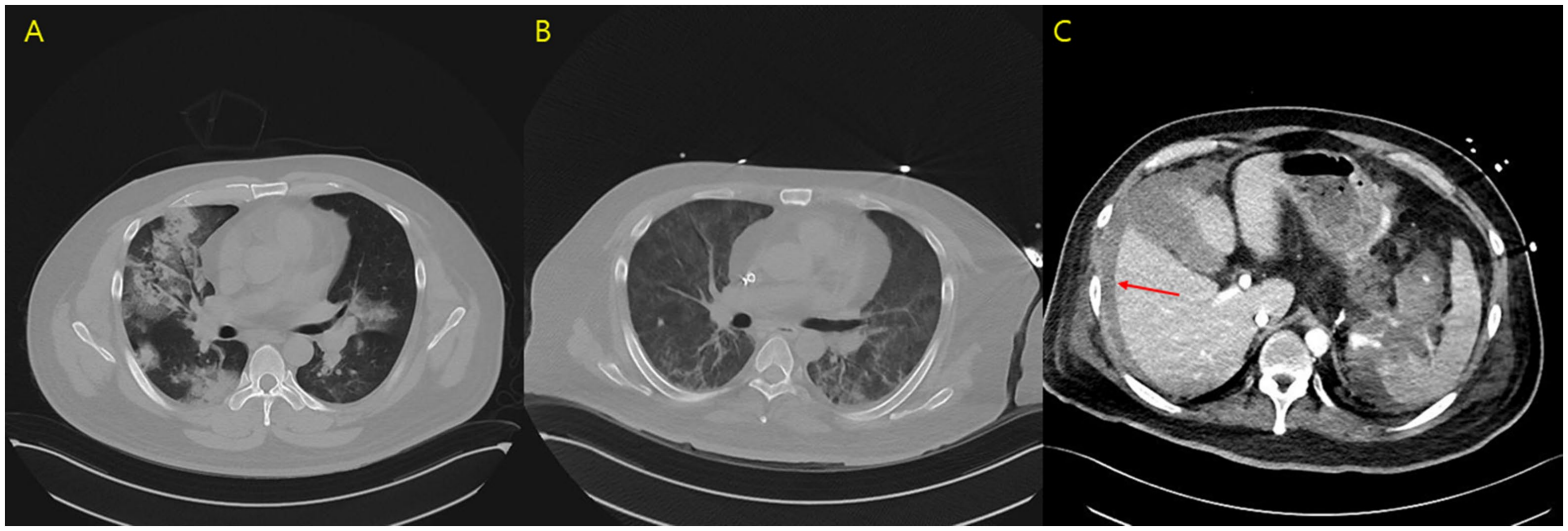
(A) Initial chest computed tomography scan. (B) Chest computed tomography scan on day 13 of hospitalization. (C) Abdominal computed tomography scan on day 13, revealing free fluid collection, hemoperitoneum (arrow), and contrast extravasation from the splenic artery.
